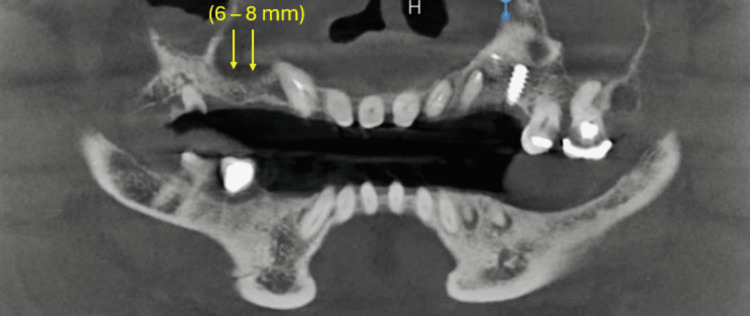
Coronal view of CBCT The yellow arrows indicate the reduced height (6-8 mm) of the alveolar ridge. CBCT, cone beam computed tomography
